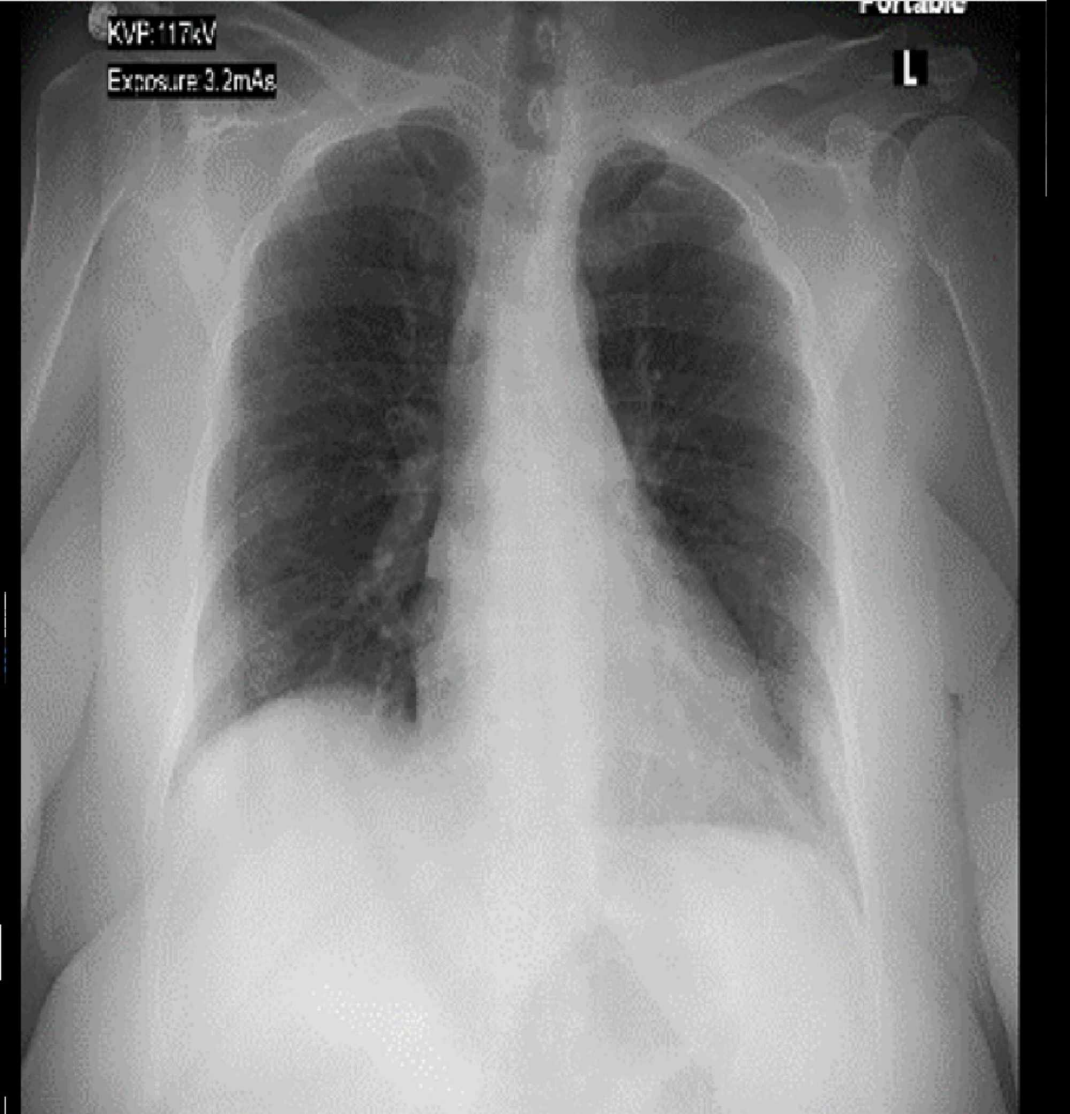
Initial X-ray Normal heart size, no mediastinal widening, no lung consolidation or effusion.
